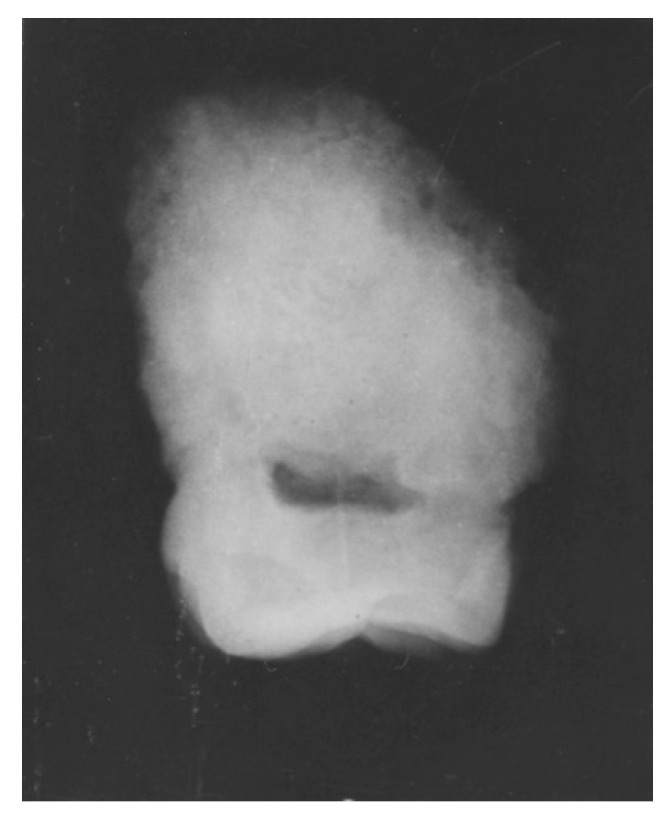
Australopithecus africanus StW Hom 2/69. Radiograph of isolated maxillary M3 crown.
